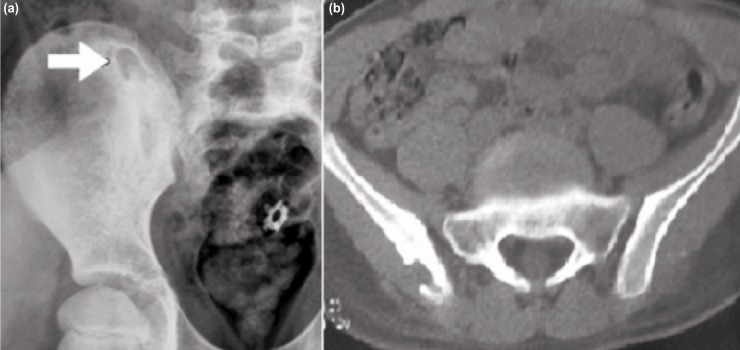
(a) Radiograph of a lytic lesion with surrounding sclerosis in the right posterior ilium and (b) CT scan showing a breach in the outer cortex of the ilium.
